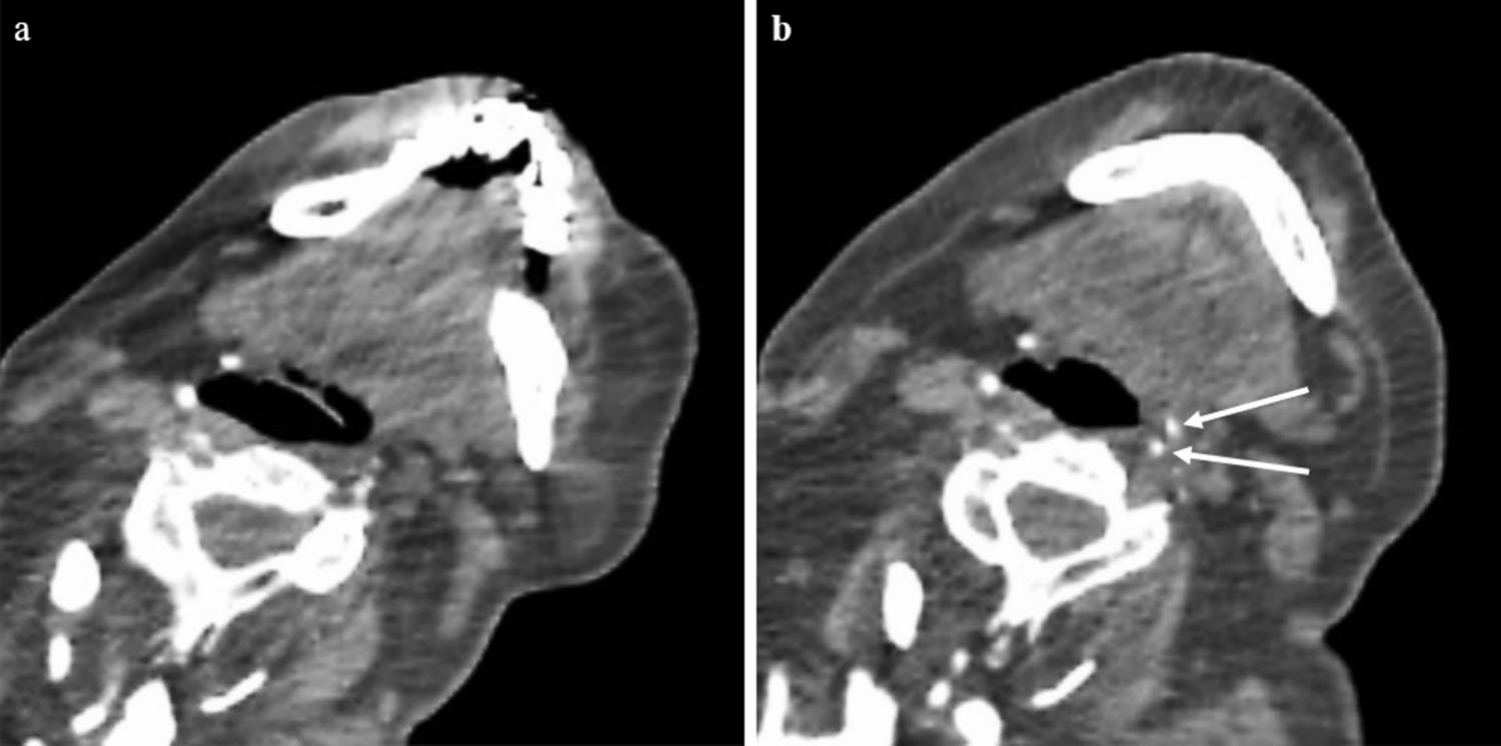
Axial computed tomography (CT) images of the FOP patient taken pre- (a) and post-COVID-19 infection (b). New foci of heterotopic ossification surounding the oropharynx at the level of cervical vertebrae No 5 (C5) appeared after COVID-19 infection convalescence (white arrows). Due to disease progression resulting in severe ambulatory restriction, movement restraint and fixed mandibula, there is a difference in neck position compared to baseline scan (CT machine*: Toshiba Aquilion Large Bore 16 slices CT scanner, Toshiba Medical Systems Corporation, Tokyo, Japan*)
